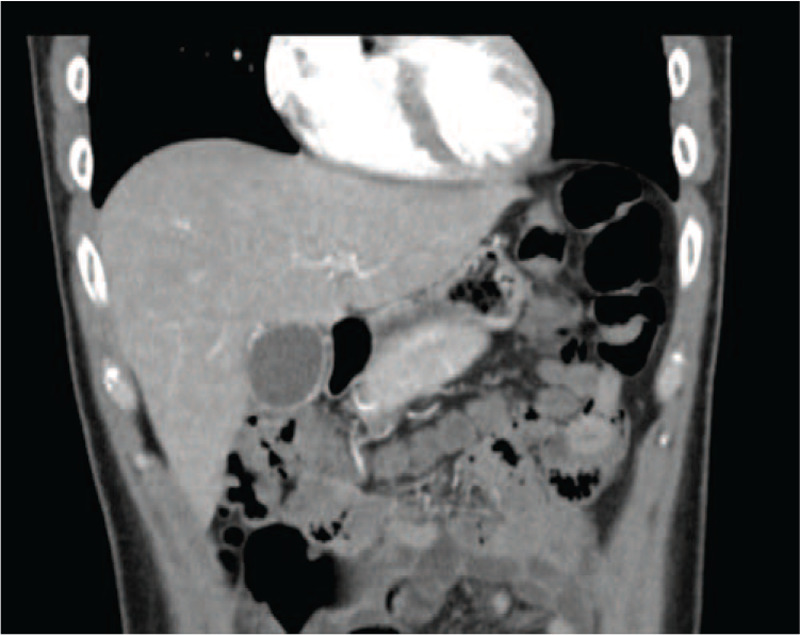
Computed tomography of the abdomen revealed a distended gallbladder with mild wall-thickening.
